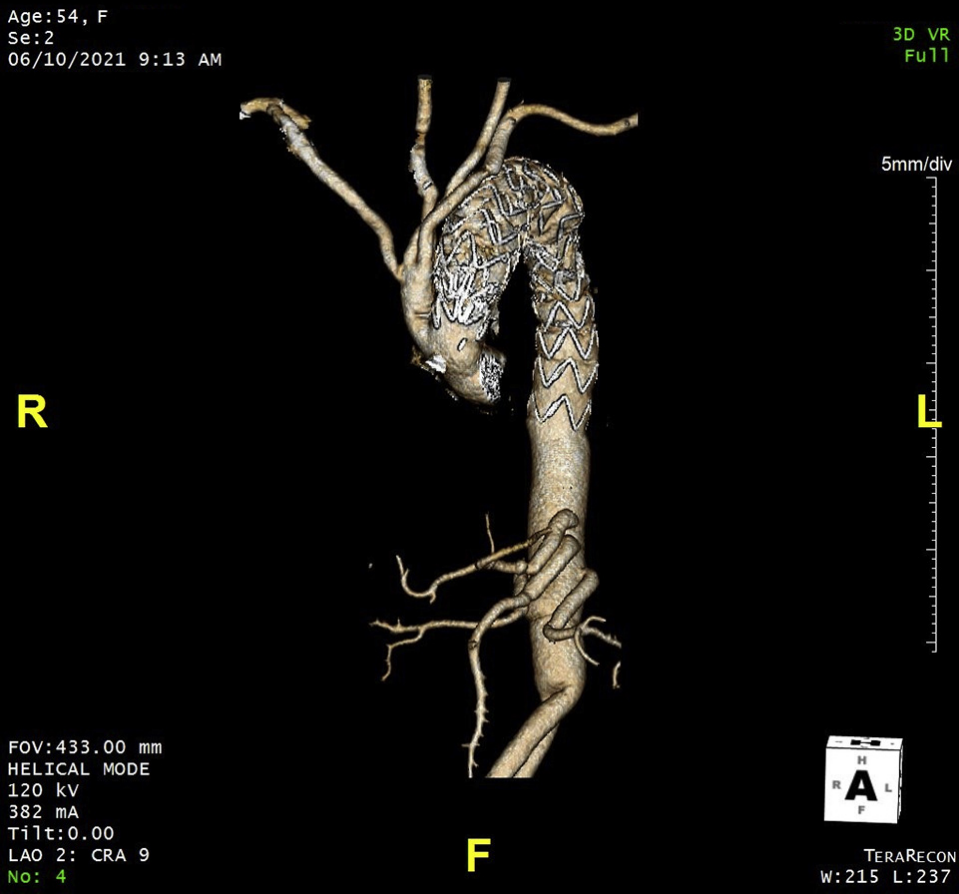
Three-dimensional computed tomography after 4-vessel arch debranching and zone 0 thoracic endovascular aortic repair (technique #1) and open replacement of the distal descending aorta to bilateral common iliac arteries.
